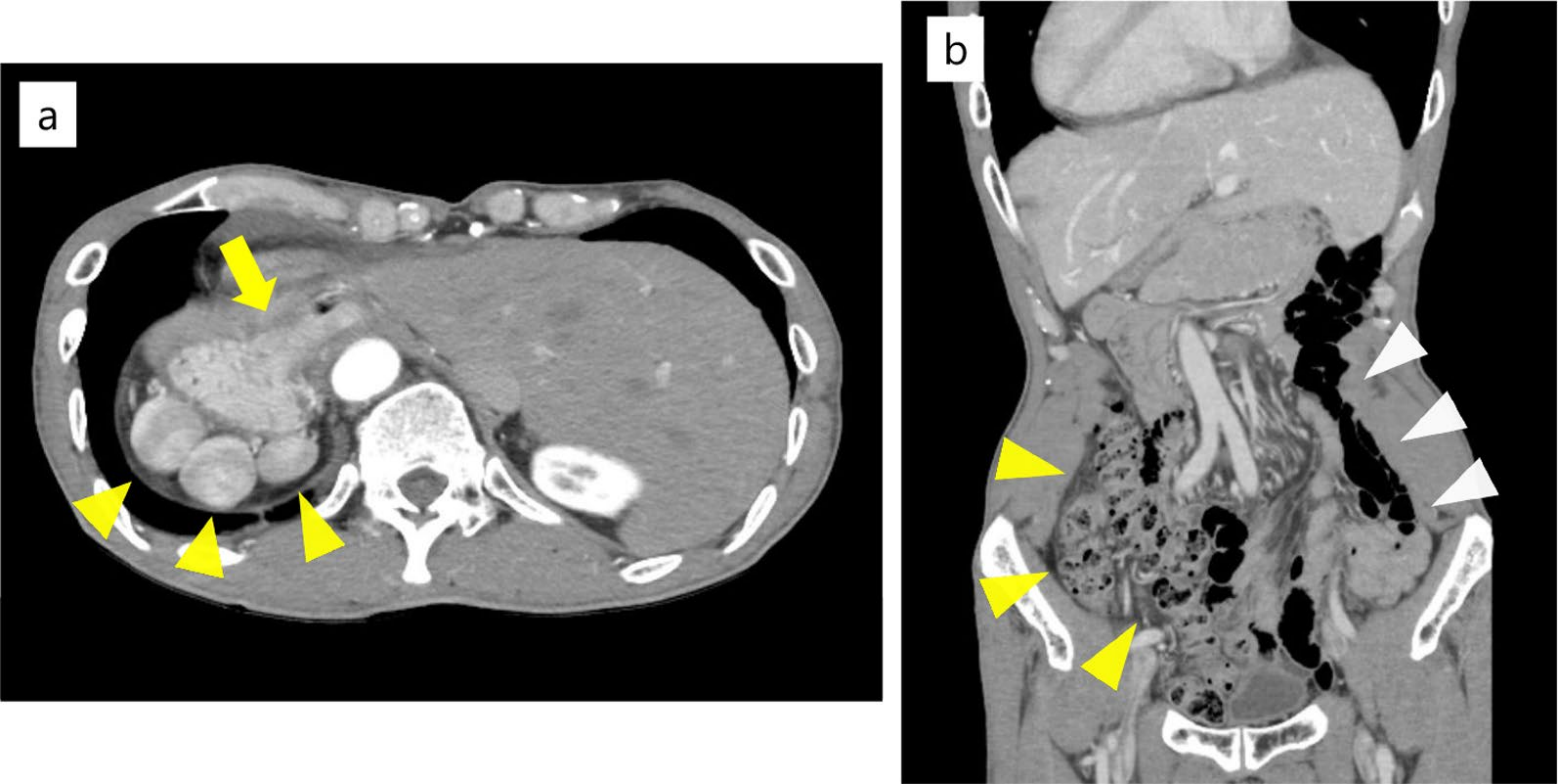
Computed tomography findings. a Situs inversus totalis, irregular thickening of the gastric wall (yellow arrow), and multiple spleens (yellow arrowheads) were observed. b The large bowel was located predominantly in the right abdomen (yellow arrowheads), and the small bowel was located predominantly in the left abdomen (white arrowheads)
